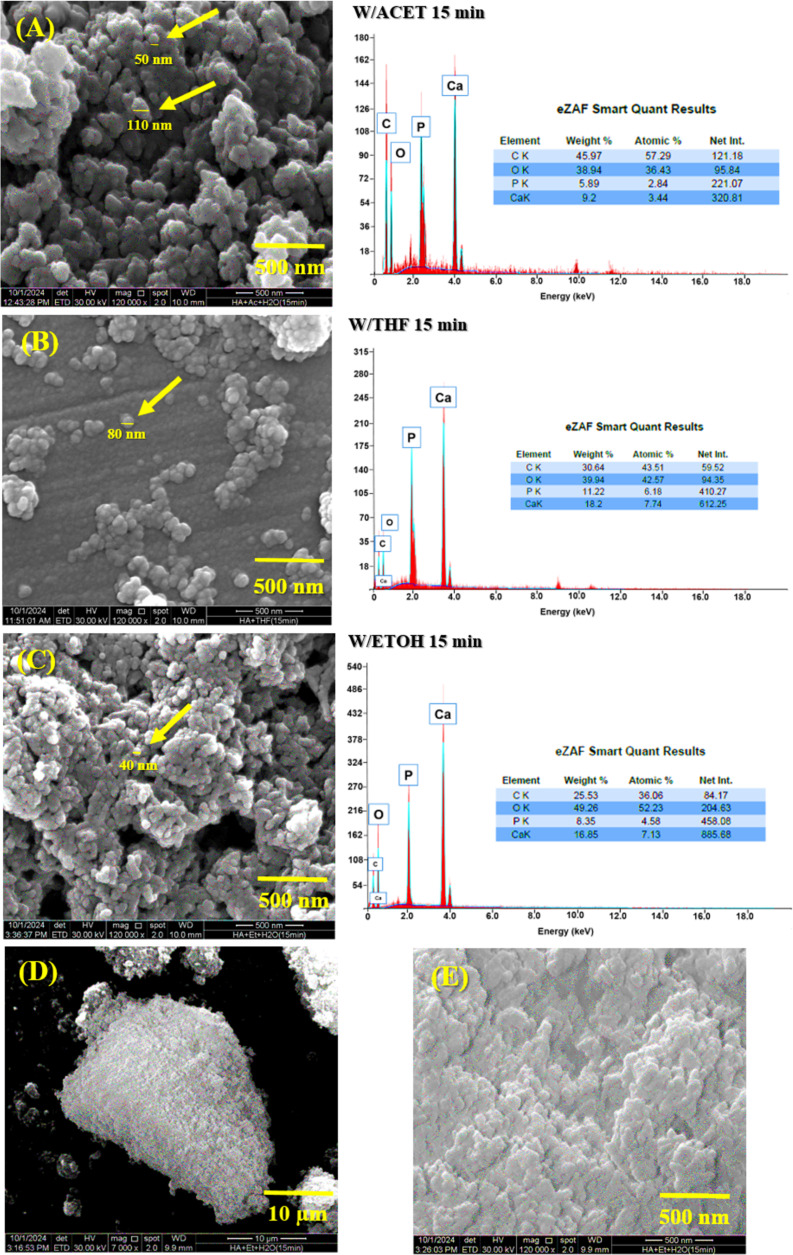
FESEM images of nHA synthesized using 15 minutes of ultrasonic irradiation: (A) W/ACET, (B) W/THF; (C–E) W/ETOH.
